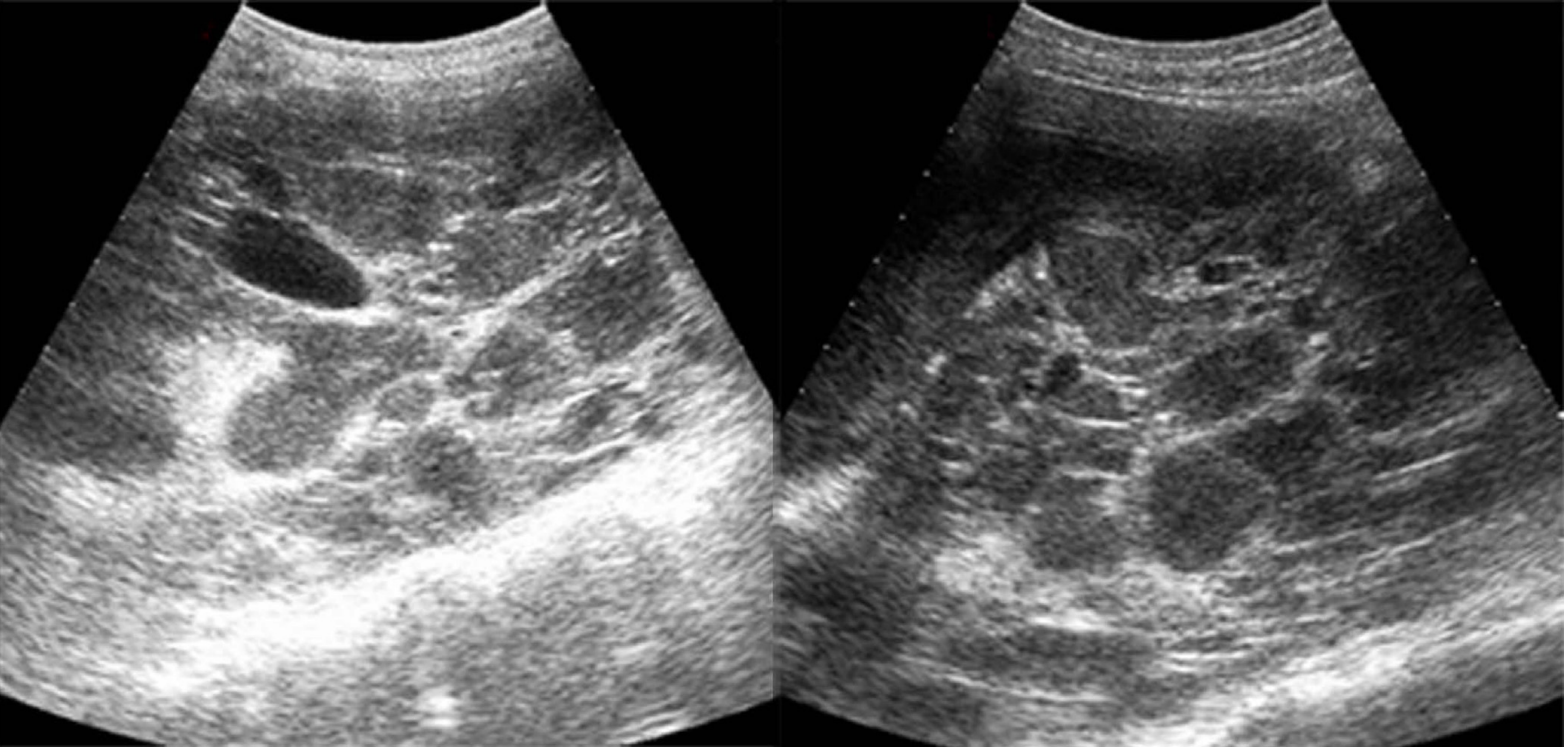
Sagittal gray-scale ultrasound scan of both kidneys obtained before intervention shows numerous cystic space-occupying lesions with marked internal echogenic debris and thick septa representing the numerous infected cysts.
